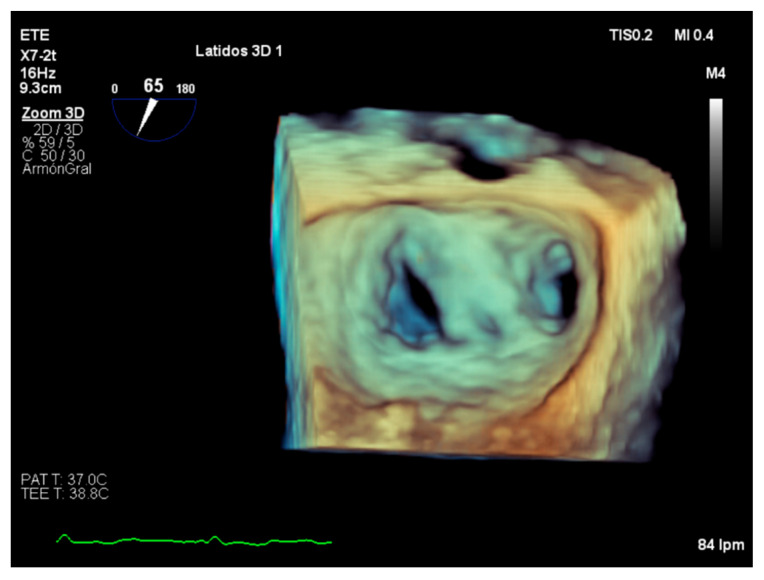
3D transesophageal echocardiography. Clips placed on the mitral valve after transcatheter edge-to-edge repair procedure.
